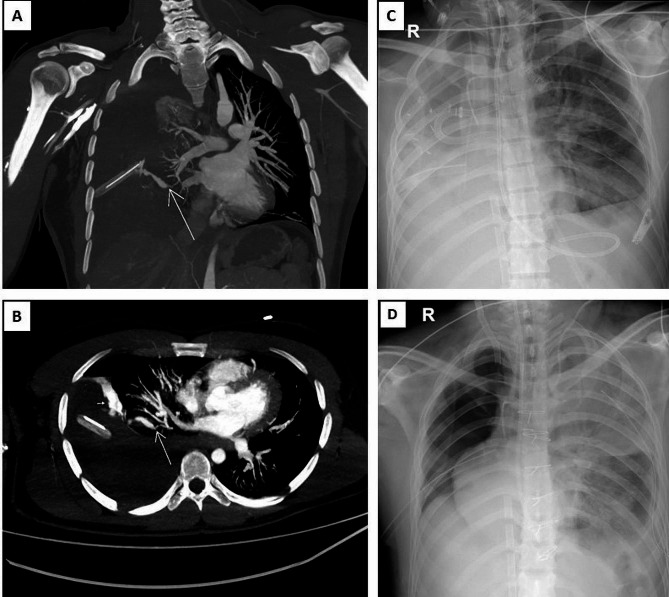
Thoracic Trauma and ECMO support. A y B. Multiplanar reconstruction with maximum intensity projection. Coronal and axial lung views showing bleeding from a posterior basal subsegmental artery of the lower right lobe (arrow) with extravasation of the contrast medium into the pleural cavity resulting in a massive coagulated hemothorax. C. Chest X-Ray shows central ECMO cannulation as a support after lung pneumonectomy. D. Chest X-Ray shows peripheral ECMO cannulation as pulmonary support due to respiratory failure
